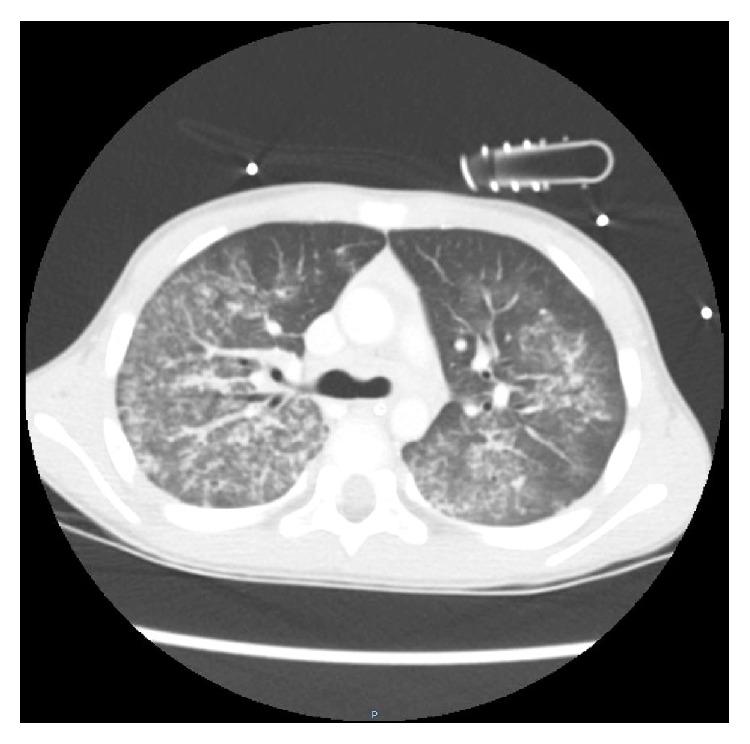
Chest computed tomography shows areas of ground-glass attenuation and a reticular micronodular appearance in both lung fields.
